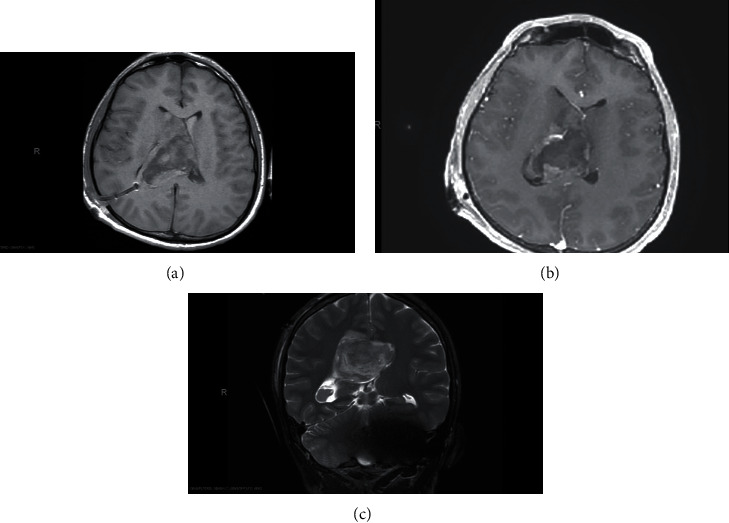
Brain MRI axial (a, b) and coronal view with contrast T2 (c).
